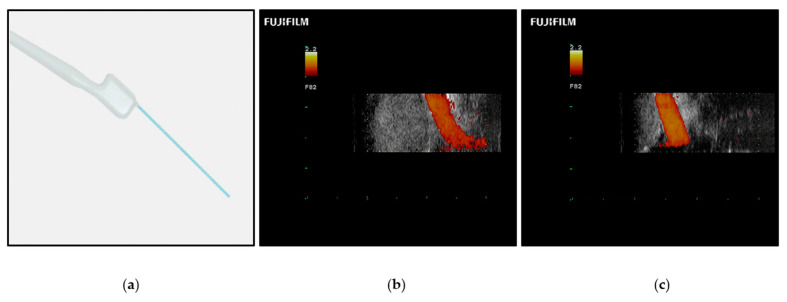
(a) Fujifilm pituitary guidance transducer (Fujifilm Healthcare Americas Corporation, Twinsburg, OH, USA). (b) Intraoperative Doppler ultrasonography showing a pituitary tumor and the cavernous segment of the internal carotid artery (ICA) before tumor debulking. (c) Intraoperative Doppler ultrasonography showing a pituitary tumor and the cavernous segment of the ICA after tumor resection. Reprinted/adapted with permission from Ref. [57]. 2022, Fujifilm Corporation.
